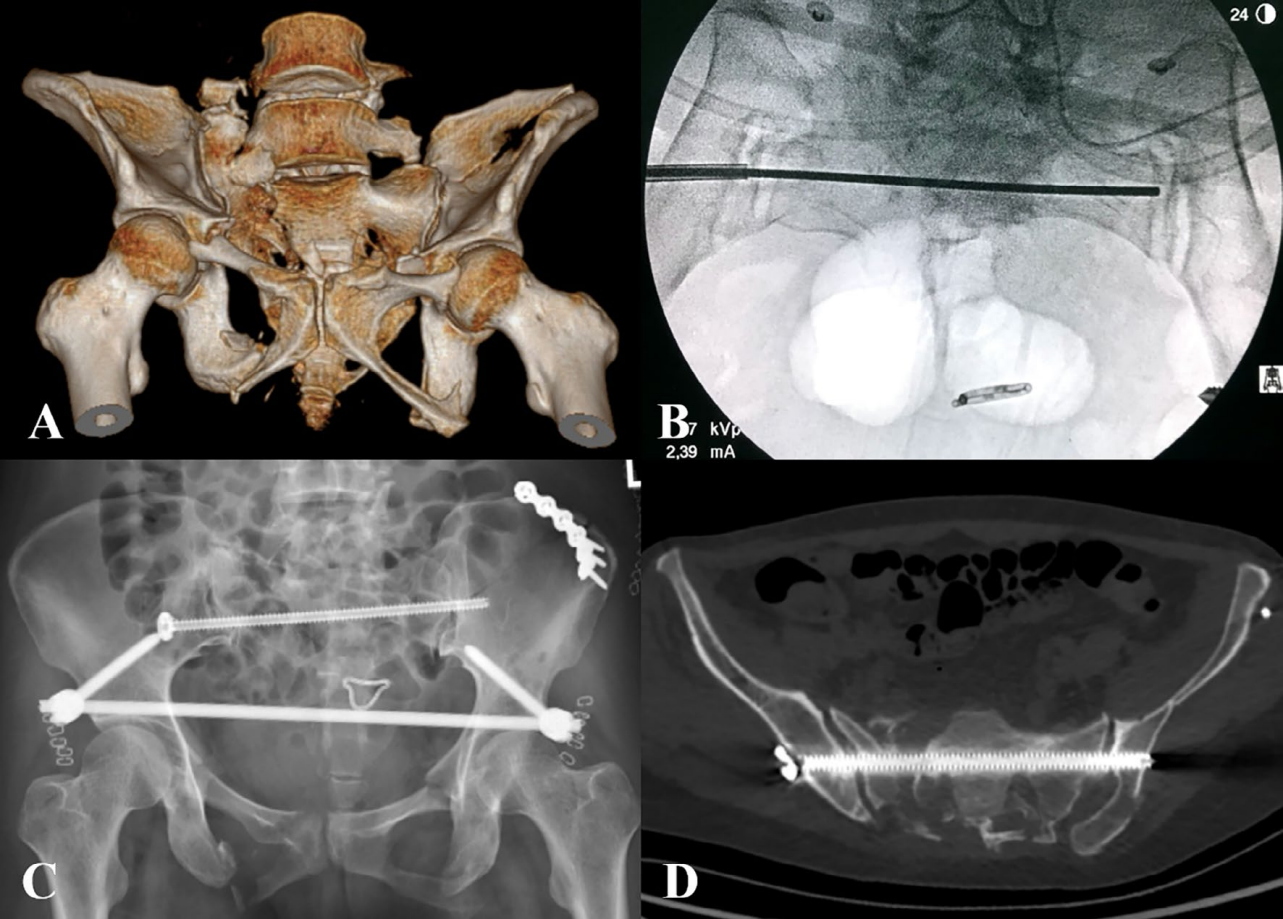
A 45‐year‐old woman suffered a displaced pelvic fracture (OTA‐61C2.2) (A). A reversed Kirschner wire was placed along the percutaneous kyphoplasty canula on the inlet view (B). Postoperative pelvic anteroposterior radiograph (C) and axial CT image showed the screw engaged the cortex of sacral nerve root tunnel (Grade‐2) (D).
